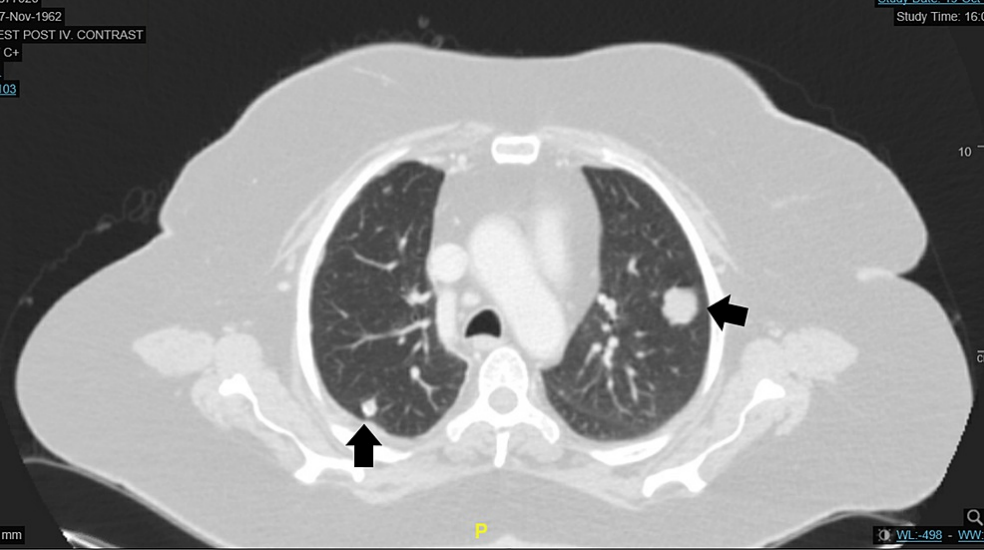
CT scan of chest showing multiple lung metastases (black arrows).
